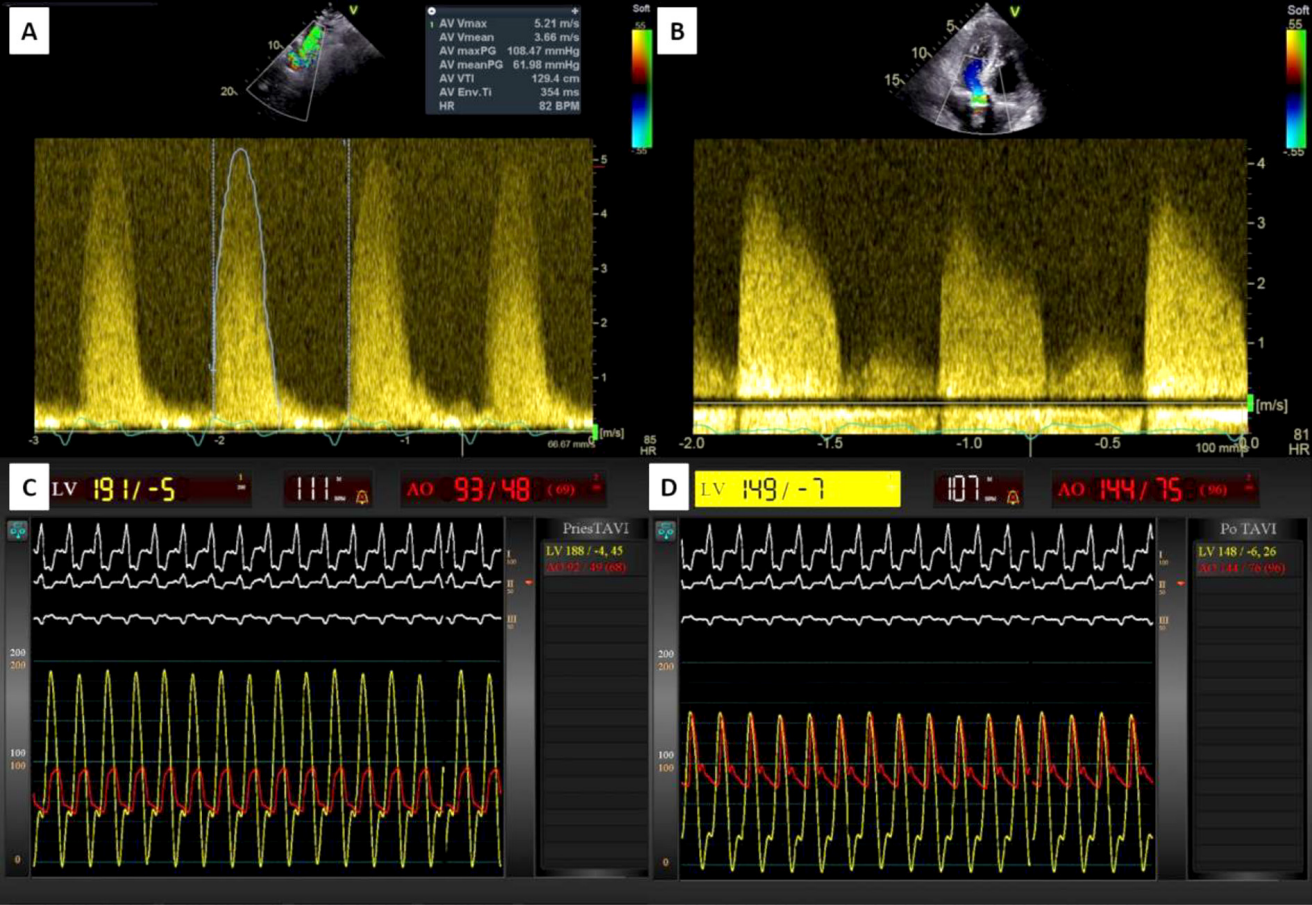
Patient hemodynamic characteristics pre- and post-TAVI. A – transoesophageal echocardiography demonstrating severe aortic valve stenosis on continuous-wave Doppler; B – transthoracic echocardiography demonstrating concomitant aortic regurgitation; C and D – aortic and ventricular pressure curves pre- and post-TAVI
